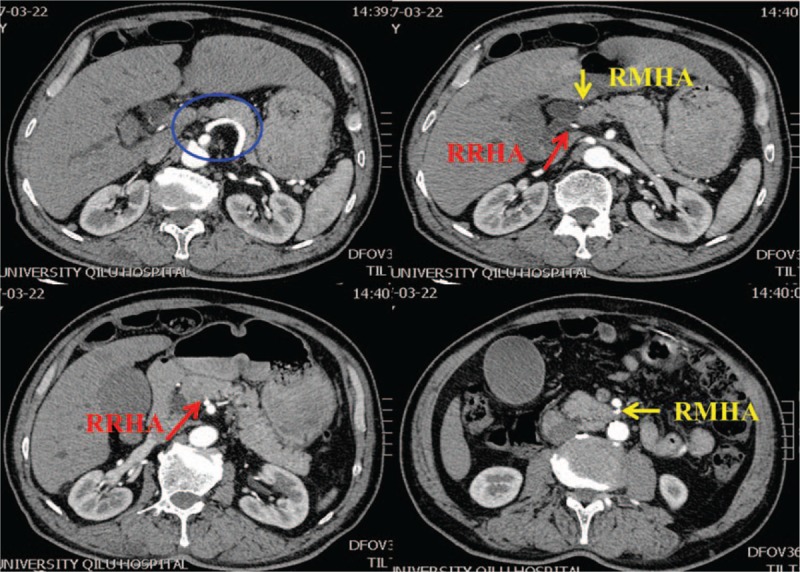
Case 1: preoperative computed tomography scan images showing aberrant hepatic arteries. A right and middle hepatic artery are arising from the superior mesenteric artery (SMA).
